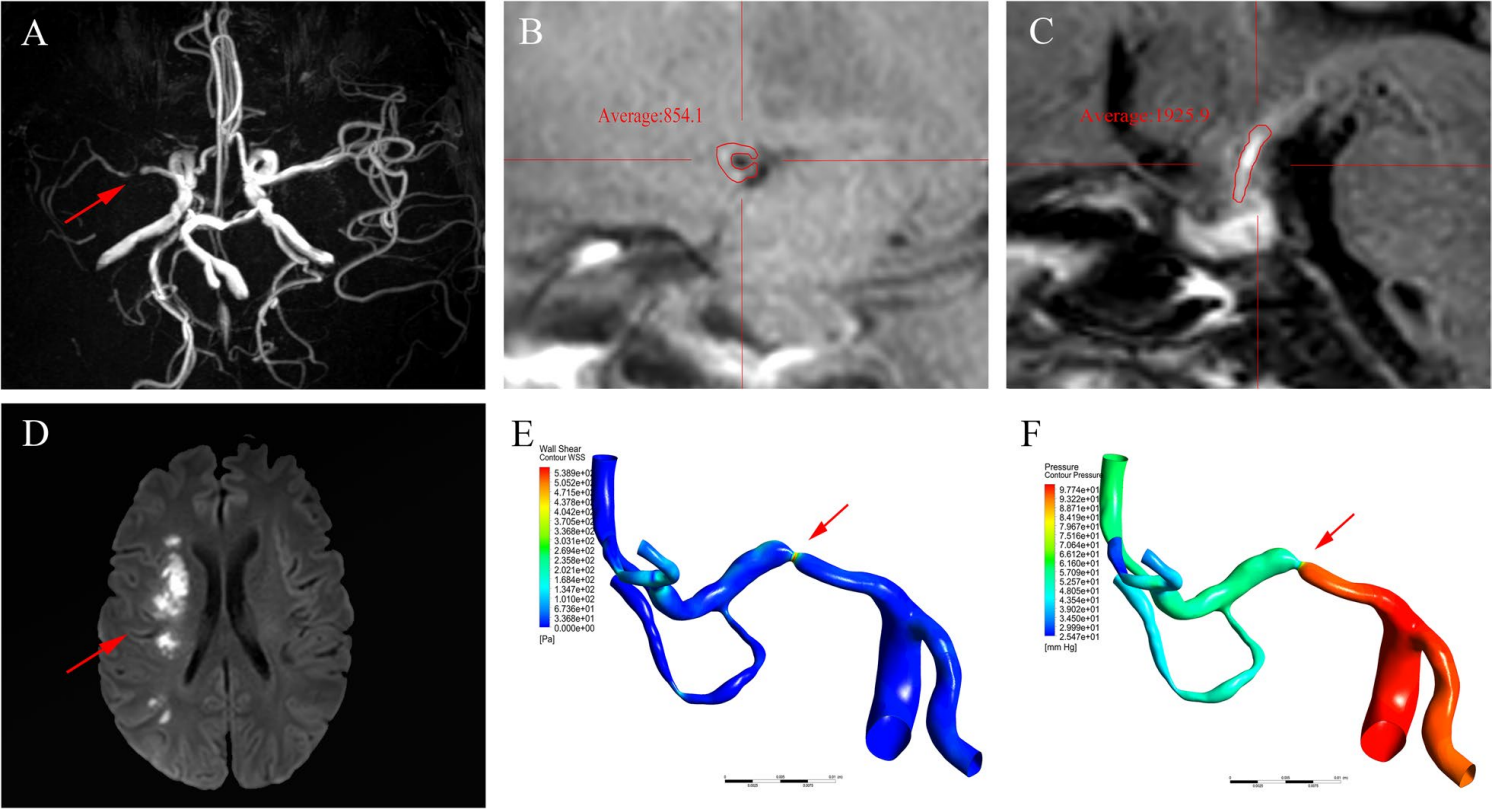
Adult male with severe stenosis of right middle cerebral artery (R-MCA). A R-MCA stenosis in MRA; B, C mean signal value of R-MCA plaque and pituitary stalk on post-contrast HR-VWI; D DWI showed acute infarction in the right cerebral hemisphere; E, F show the results of WSS and pressure contour maps, respectively
